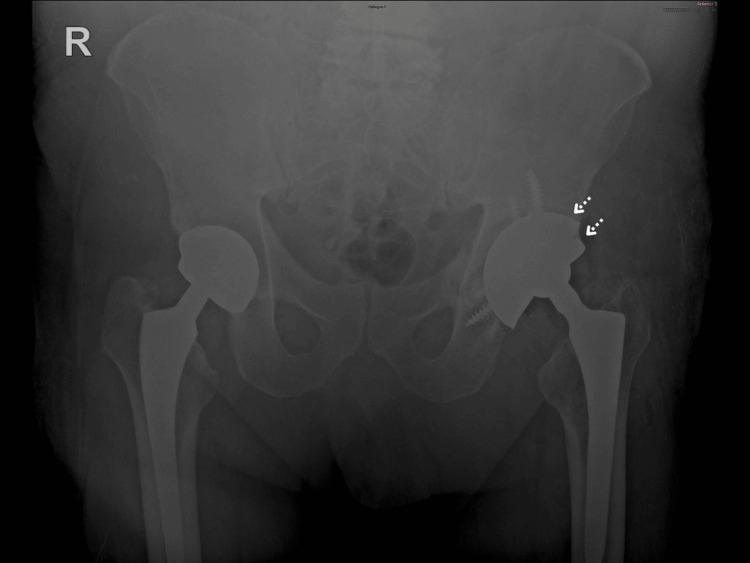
Immediate postoperative AP pelvic radiograph showing correct positioning of the double-cup construct and restoration of hip biomechanics.
